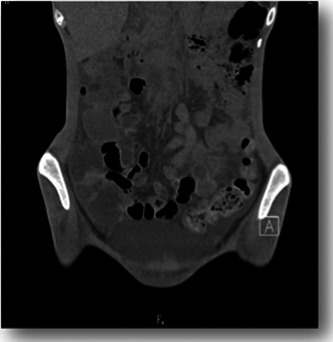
Abdominal computed tomography (longitudinal view). There is a thickening of the intestinal wall that is suggestive of intestinal edema.
